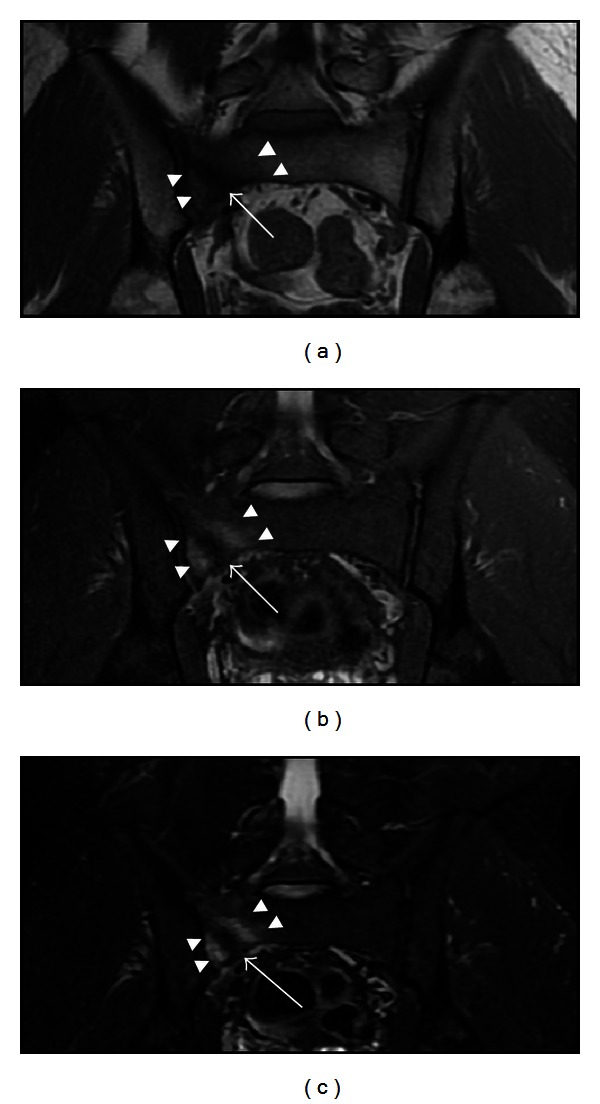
The coronal T1-w (a), STIR (b) and fat-suppressed T2-w (c) MR images of the pelvis depict an area of bone marrow edema in the right sacral ala (arrowheads) surrounding a low signal intensity obliquely oriented vertical fracture line (arrow).
